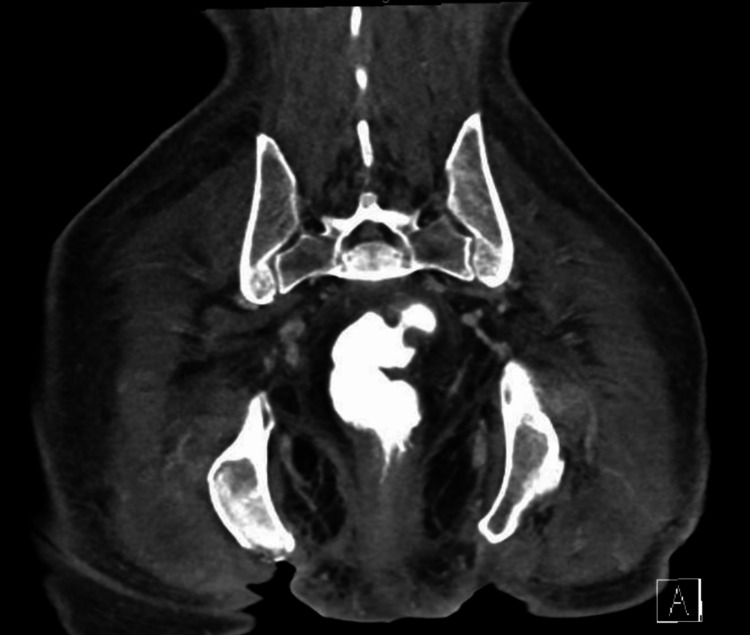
Coronal CT pelvis with contrast demonstrating osteomyelitis of the right ischial tuberosity
